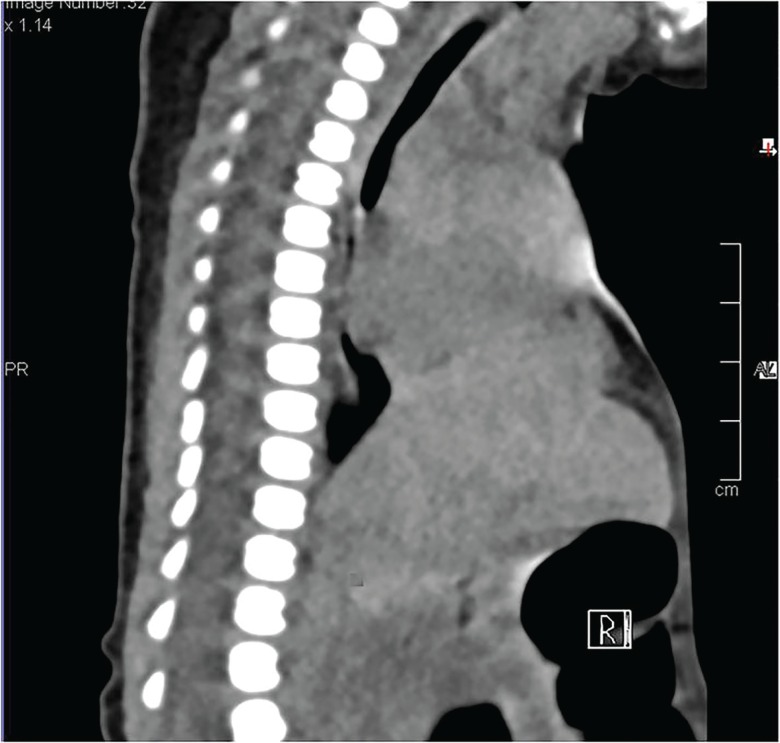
CT scan of chest – Sagittal view. Note the superior defect of the sternum
